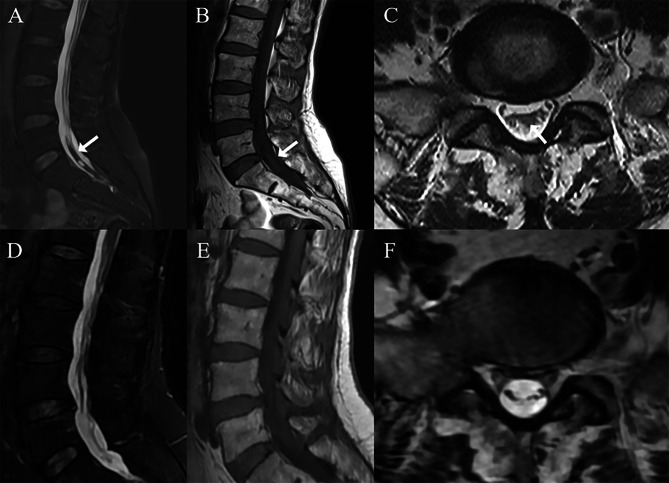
Magnetic resonance imaging (MRI) showed diffuse iso-signal intensity on T1-weighted images (B, arrow), and a low signal intensity on T2-weighted images (A + C, arrow) in the spinal canal at the level of L5 to S2, which suggested early subacute hemorrhage in the spinal subarachnoid space. Follow-up MRI (D-F) at one month revealed complete resolution of the hemorrhage
